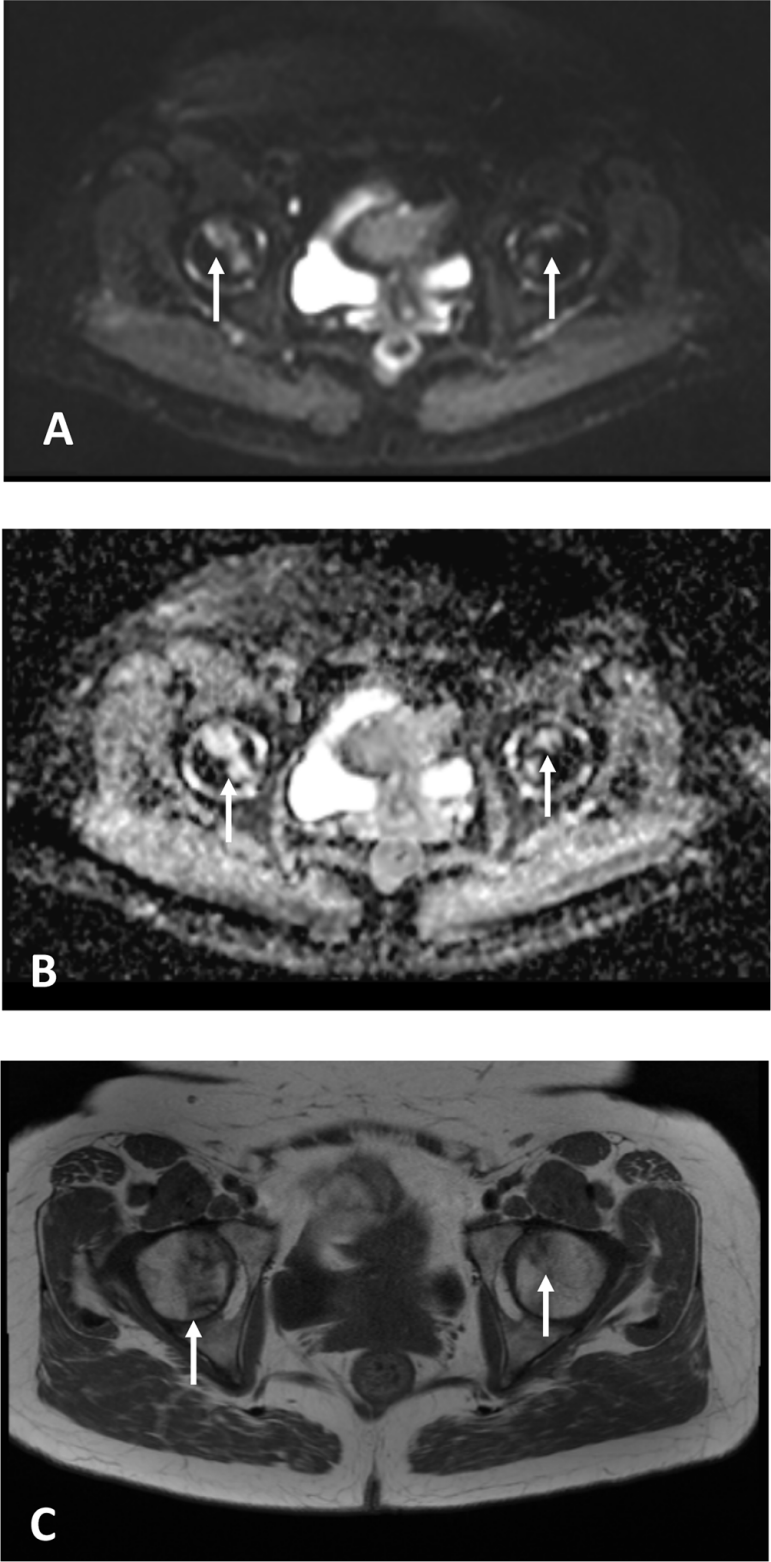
Axial MRI images (A: b50 diffusion-weighted MRI, B: corresponding apparent diffusion coefficient map, C: *T*_1_W MRI) of a 52-year-old lady on thalidomide and dexamethasone maintenance therapy. Bilateral subchondral signal changes (arrows) are evident on the diffusion weighted MRI (A, B) and on *T*_1_W MRI (C). No femoral symptoms were reported at the Mme of imaging which was performed for back pain. LeP hip replacement was performed 2 years following on from this scan due to pain.
